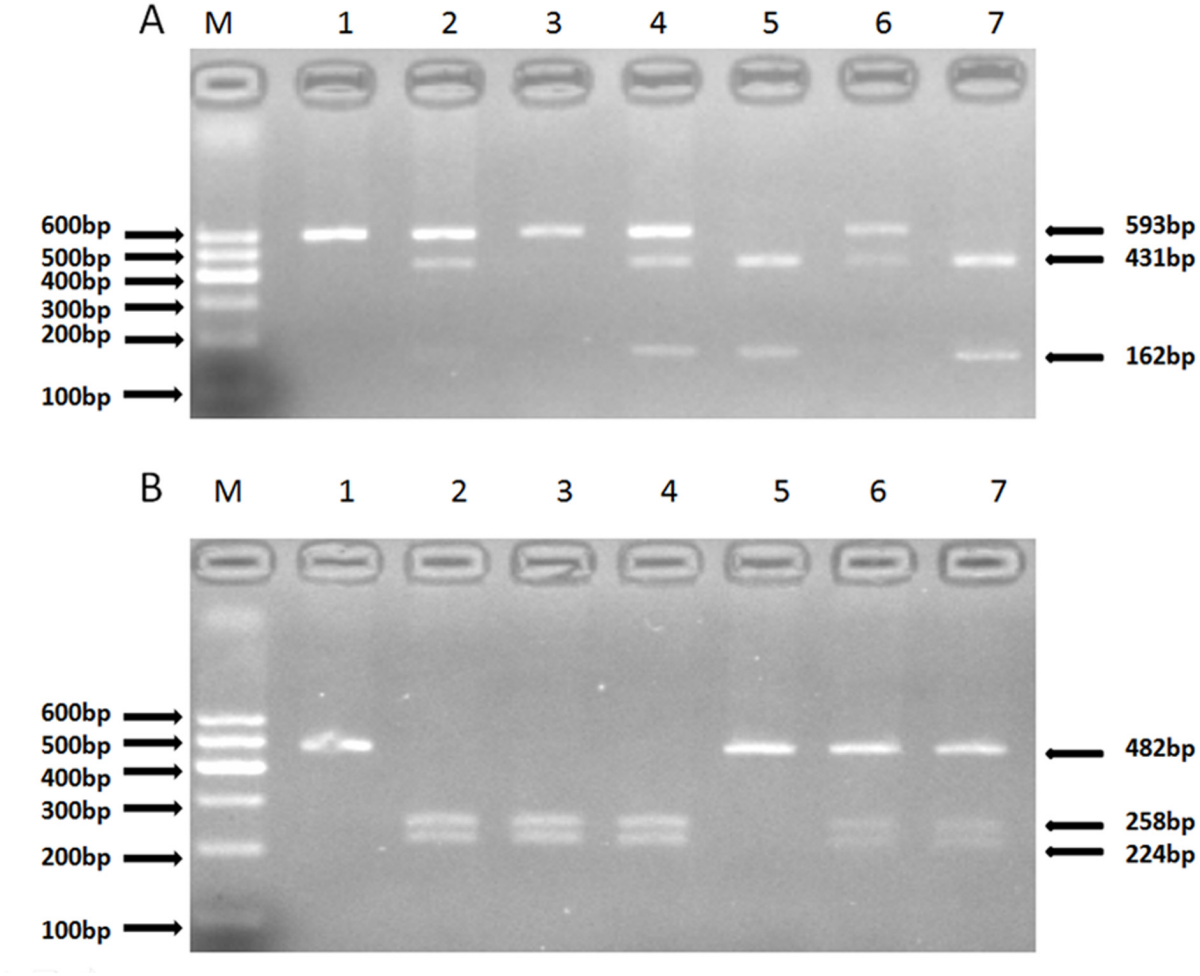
The restriction fragment length polymorphism analysis to determine the genotype. A. For rs1800871, the TT genotype shows one 593-bp band (1 and 3); the CC genotype shows two bands at 431 bp and 162 bp (5 and 7); and the CT genotype shows three bands at 593 bp, 431 bp and 162 bp (2, 4 and 6). B. For rs1800872, the CC genotype shows one 482-bp band (1 and 5); the AA genotype shows two bands at 258 bp and 224 bp (2, 3 and 4); and the AC genotype shows three bands at 482 bp, 258 bp and 224 bp (6 and 7).
